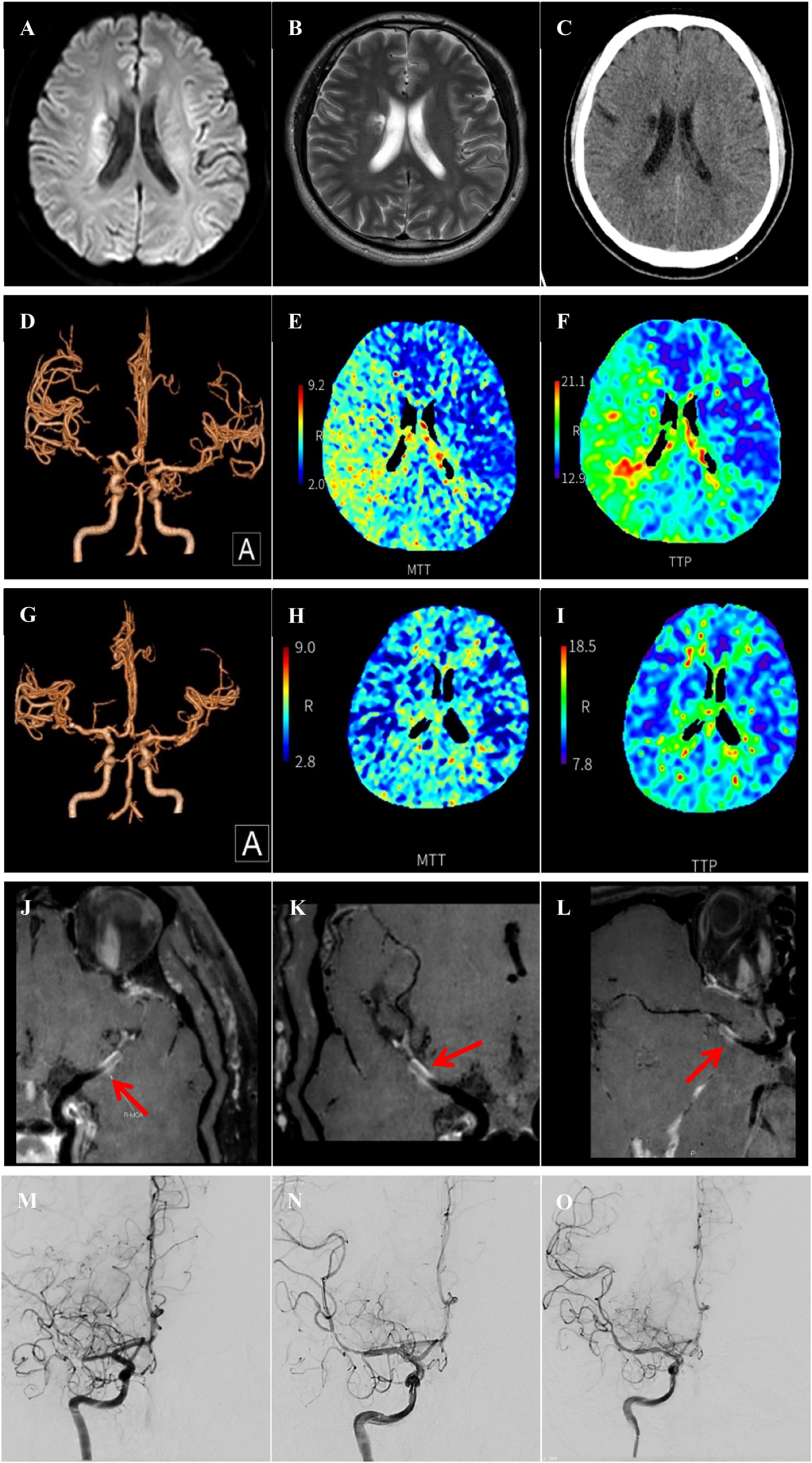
A case of sICAS in right MCA. (A–C): New infarction of right paraventricular white matter was observed in MRI and CT. (D–F): Preoperative CTA and CTP showed that M1 of the right MCA was nearly occluded and the brain tissue it innervates was hypoperfused. (G–I): Postoperative CTA and CTP showed that almost normal blood flow was restored to the M1 of the right MCA, and the corresponding cerebral tissue hypoperfusion was effectively improved. (J–L): Preoperative VW‐HRMRI indicated that the lumen of M1 of the right MCA was almost occluded, but a portion of the true lumen of the vessel wall remained, making it a candidate for angioplasty and stenting. (M–O): Preoperative DSA showed that the lumen of M1 of the right MCA was nearly occluded, and the distal branches could not be visualized. After angioplasty, a Neuroform Atlas stent was successfully implanted in the M1 of the right MCA, and follow‐up angiography 10 min later showed good blood flow. CT, computed tomography; CTA, computed tomography angiography; CTP, computed tomography perfusion; DSA, digital subtraction angiography; MCA, middle cerebral artery; MRI, magnetic resonance imaging; sICAS, symptomatic intracranial atherosclerotic stenosis; VW‐HRMRI, vessel wall high‐resolution magnetic resonance imaging.
